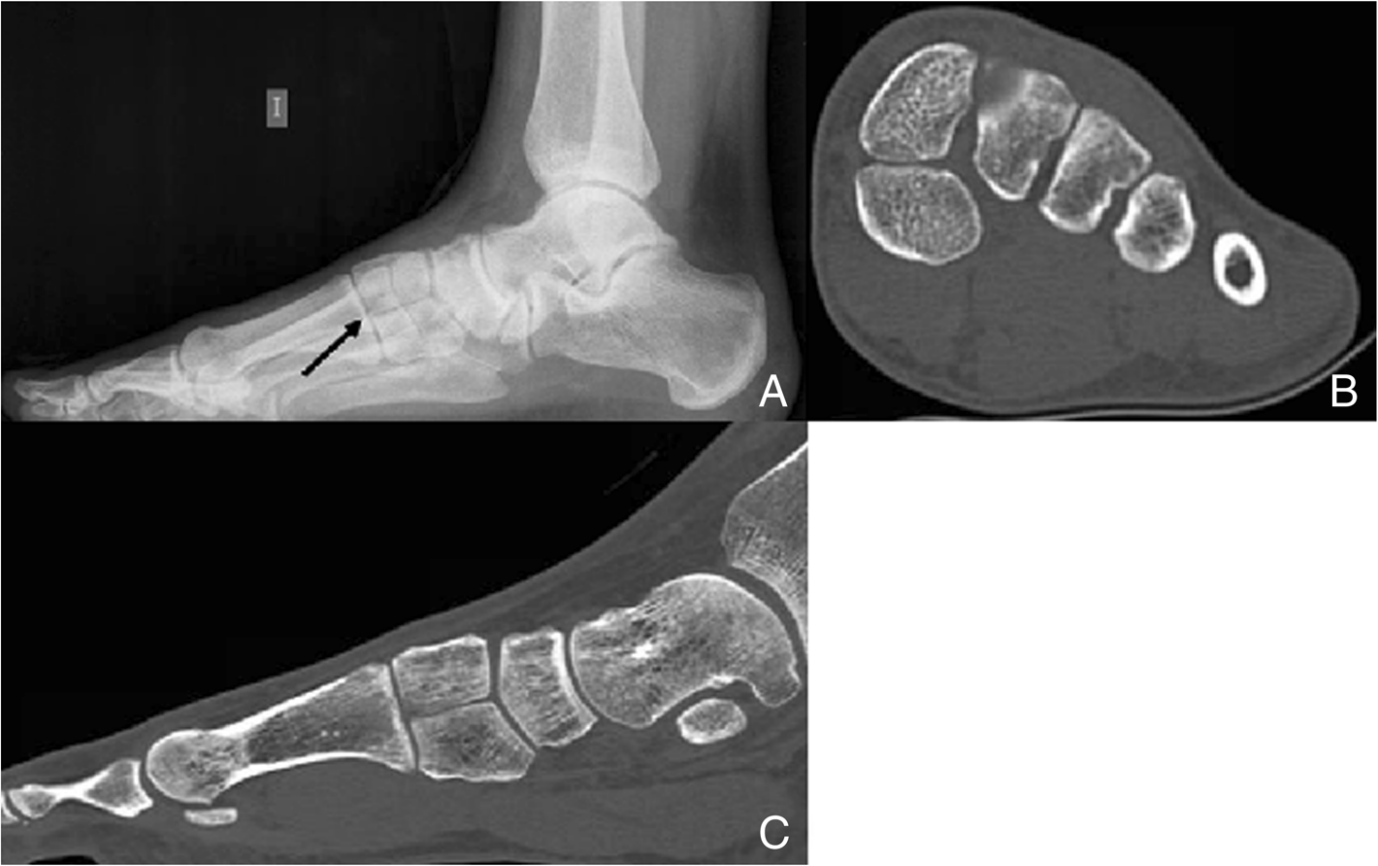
Bipartite medial cuneiform. A 32-year-old man, on work up for left flat foot. Bilateral foot radiographs were taken. a Left lateral view demonstrates a bipartite cuneiform (finding was bilateral but only right foot shown). The black arrow marks the synchondrosis in between medial cuneiform components. b CT coronal reconstructions show a slightly larger plantar cuneiform. c Sagittal CT reconstruction shows that the addition of the volume of the two bones is larger than a normal medial cuneiform would be
